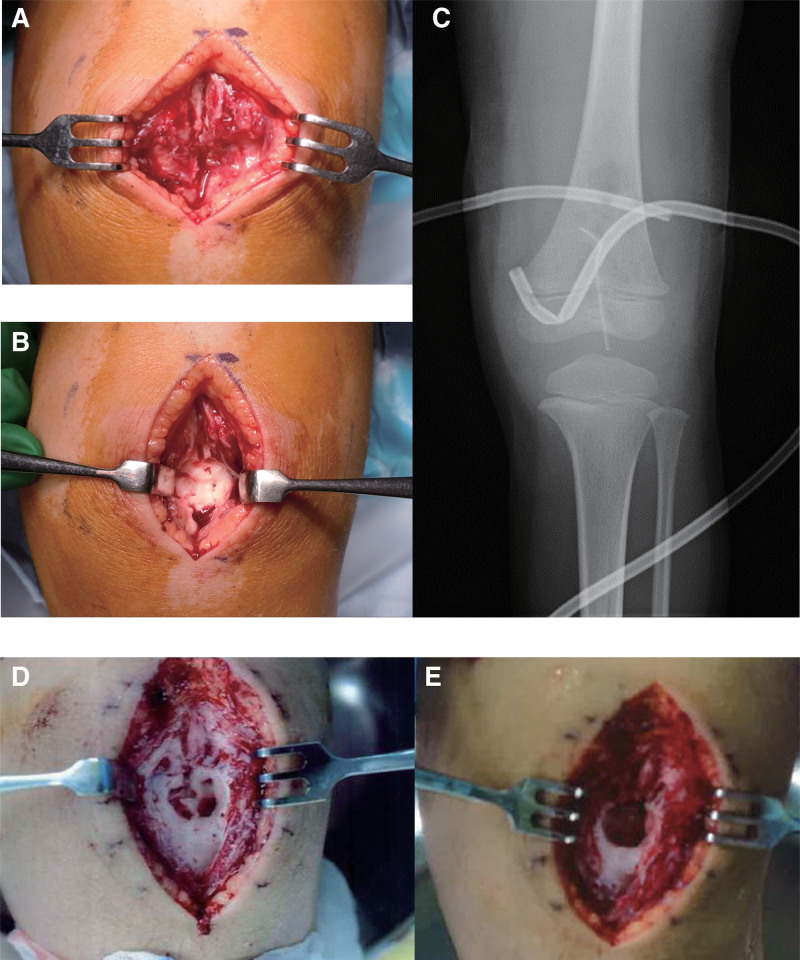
Intraoperative photographs of the left knee. Front view photographs taken before (A) and after (B) curettage of the patellar lesion at the time of the initial debridement. (C) Radiographs made immediately after the initial debridement. Note 2 drainage tubes placed in the suprapatellar pouch for postoperative continuous closed irrigation. Front view photographs taken before (D) and after (E) curettage of the patellar lesion at the time of the second debridement.
